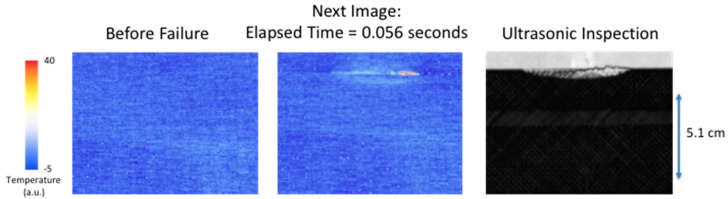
Comparison of instantaneous surface heating to ultrasonic inspection.
